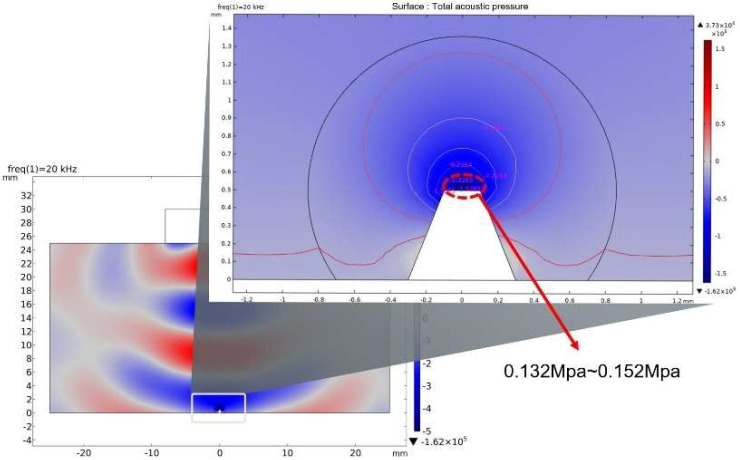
Acoustic pressure distribution of local ultrasonic cavitation field.
